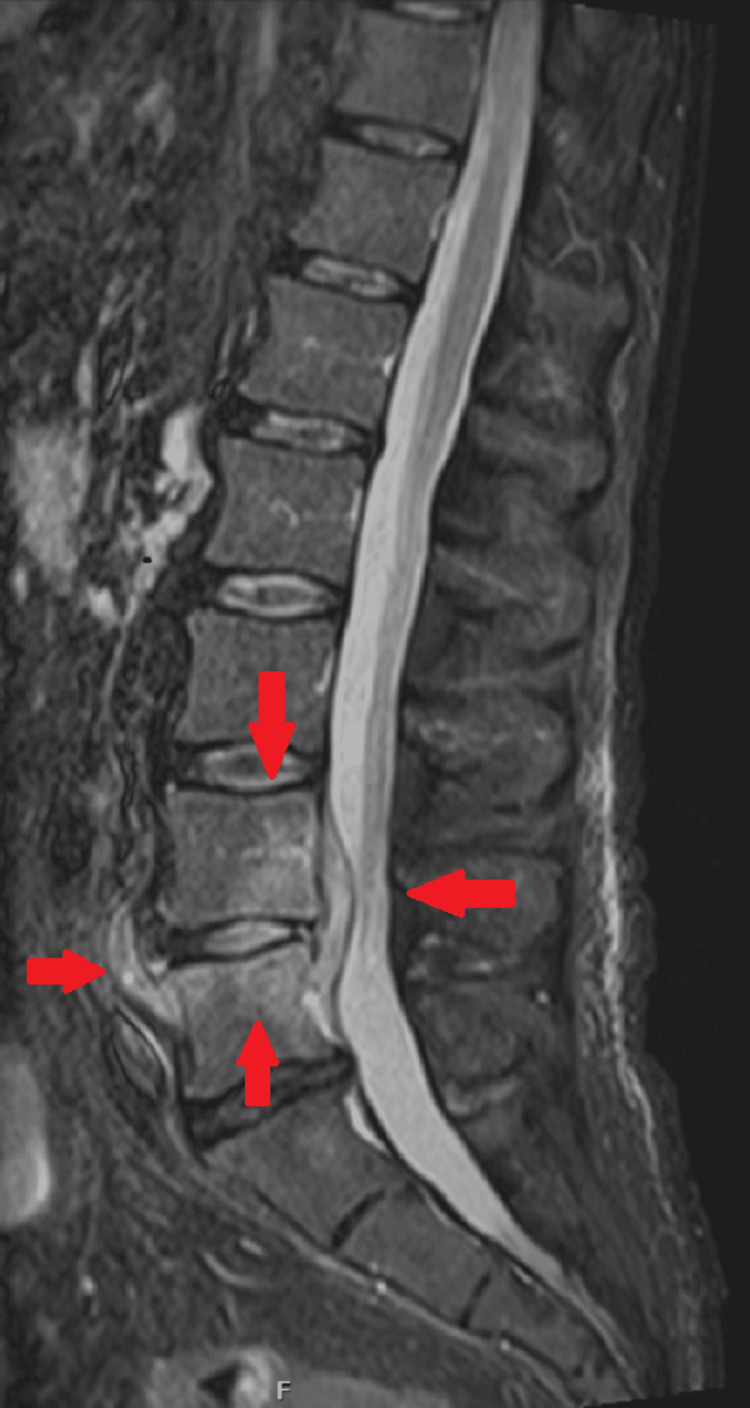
Initial lumbosacral MRI (STIR, sagittal) showing edema in the L4 and L5 vertebral bodies and an epidural abscess STIR, short tau inversion recovery
